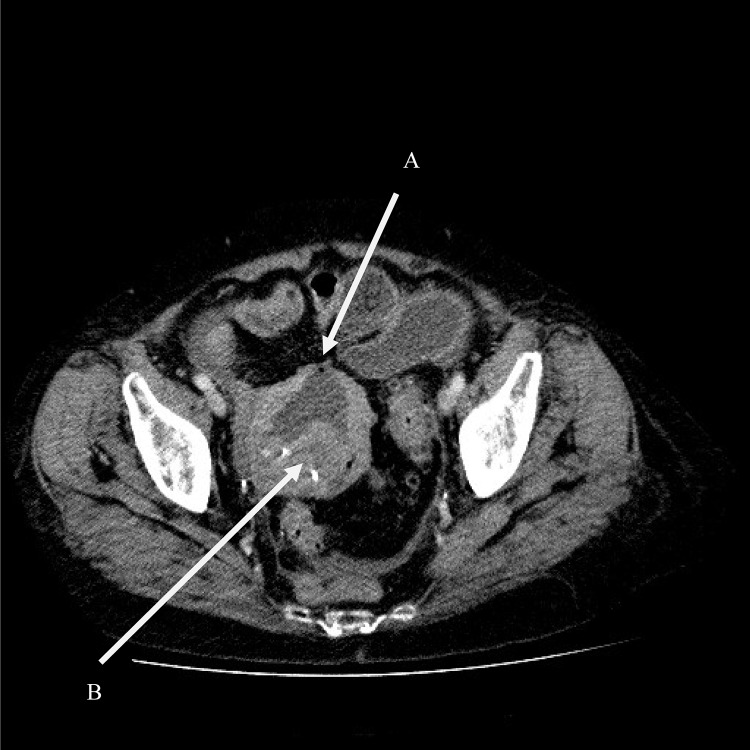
Axial computed tomography showing a fluid filled uterus with a perforation and free air in the anterior wall (A). Leiomyomata can also be seen on the posterior wall (B).
